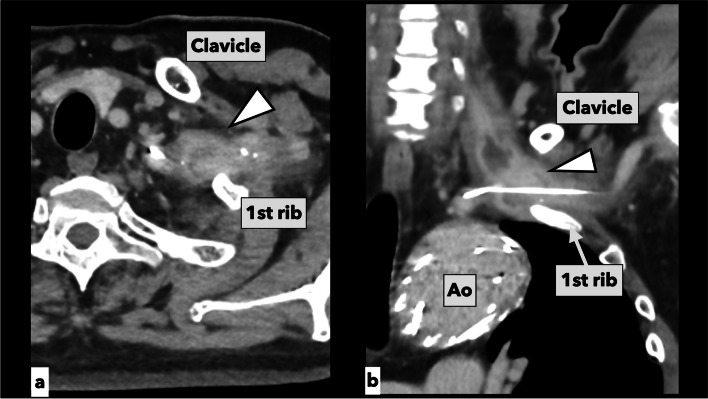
Enhanced computed tomographic image before antibiotics therapy in case 2. a Axial view of the abscess formation around the left subclavian artery and the guidewire penetrating the vessel wall of the left subclavian artery (white arrowhead). B Coronal view of the abscess formation (white arrowhead). Ao, aorta
